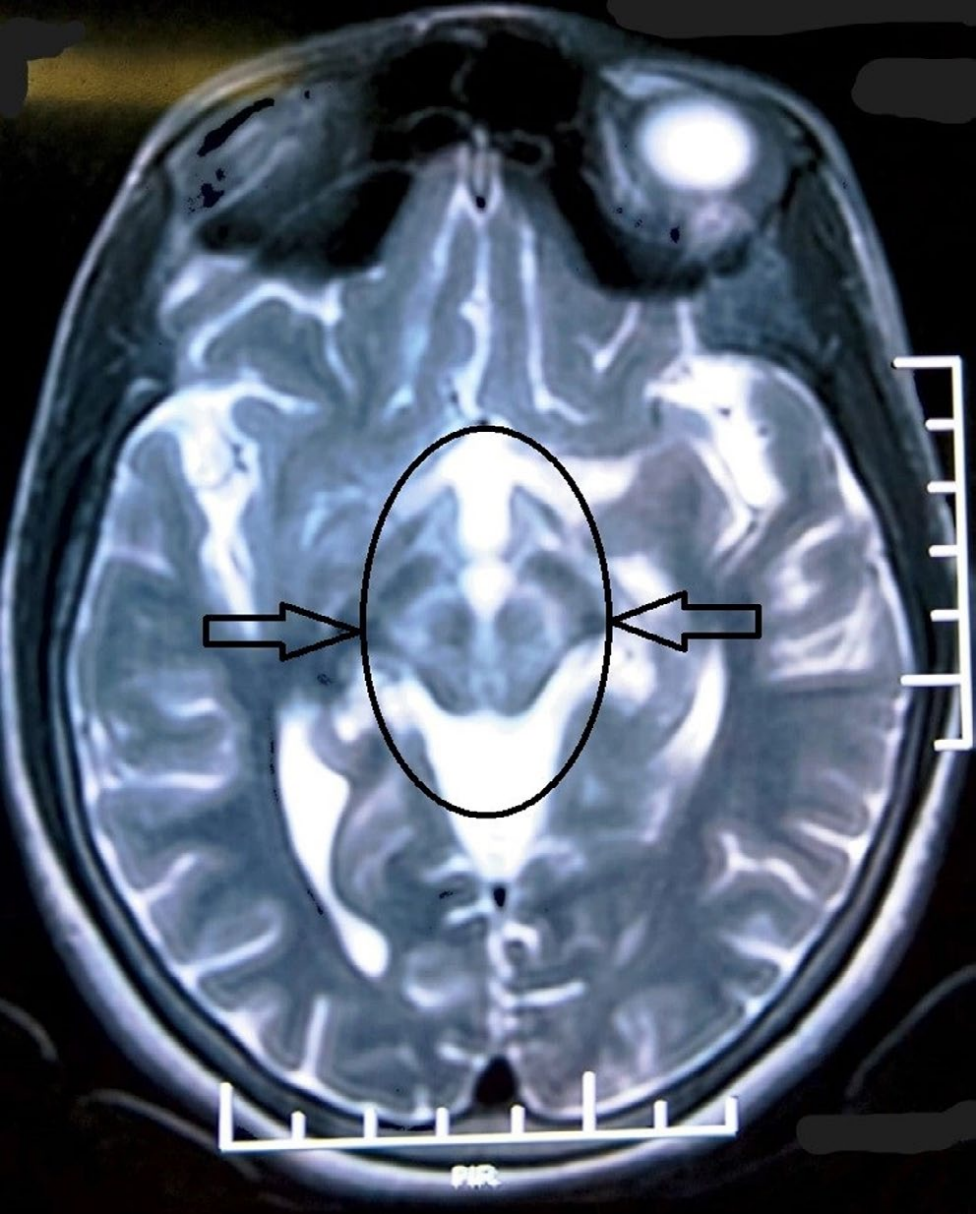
“Giant panda” sign is noted in the midbrain on MRI.
